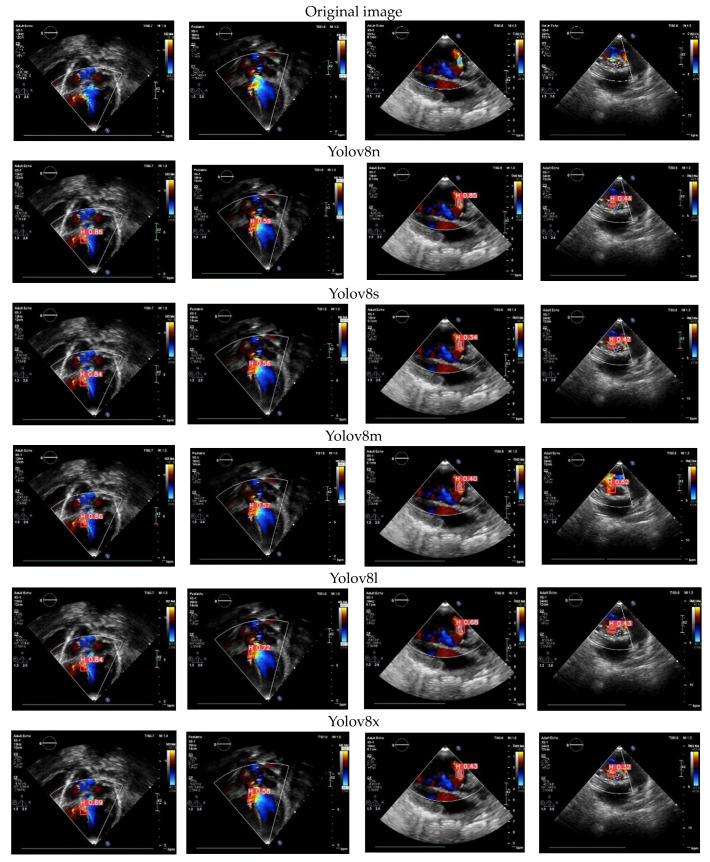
The proposed model of CSD detection in color Doppler echocardiography case.
